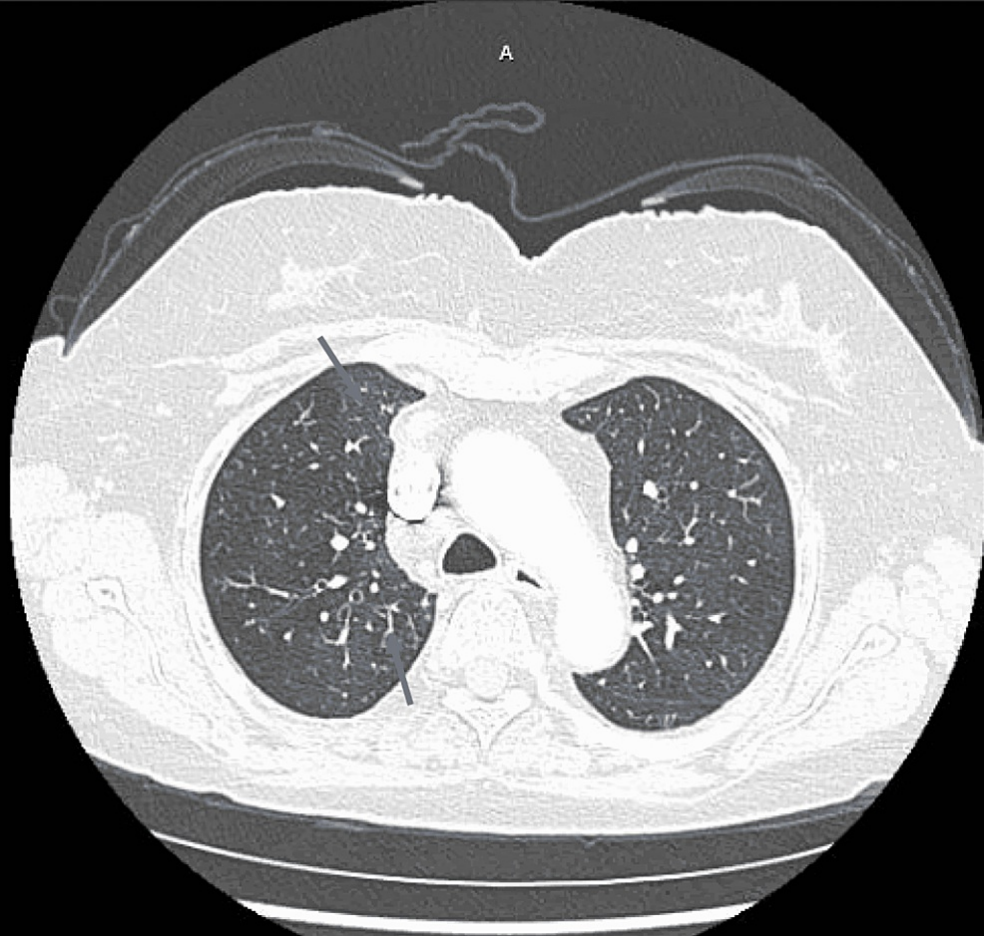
CT angiography showing diffuse patchy opacities in the right lung
